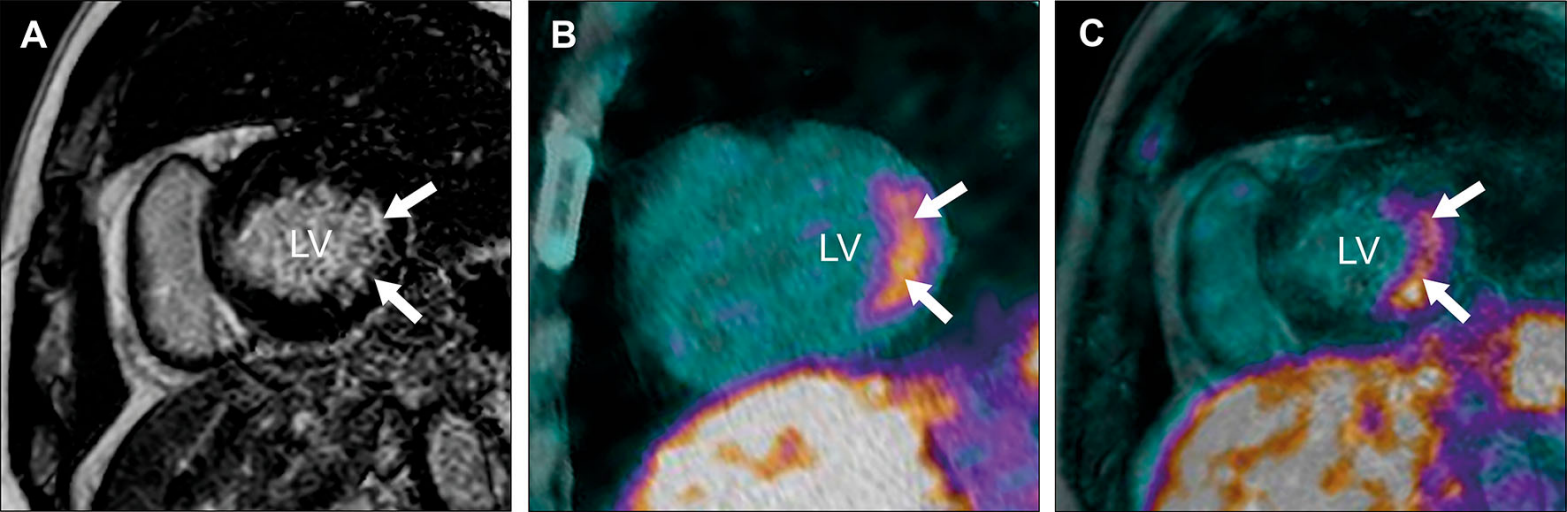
CMR revealed the presence of inferolateral subendocardial delayed enhancement in the basal part of the left ventricle (LV) in the short-axis projection (A). In the same region, ^68^Ga-DOTA-TOC PET/CT showed pathologic myocardial uptake (B). Image fusion of the ^68^Ga-DOTA-TOC PET and CMR confirmed the colocalization of both findings (C). White arrows indicate pathologic findings
